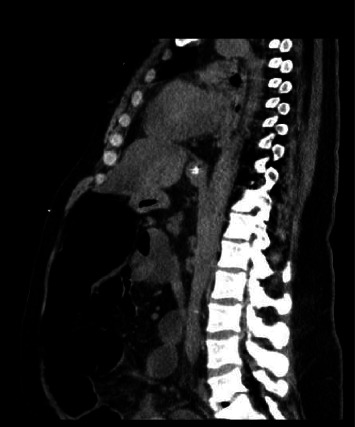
CT scan of the abdomen showing diffuse dilatation of the colon without evidence of obstruction.
